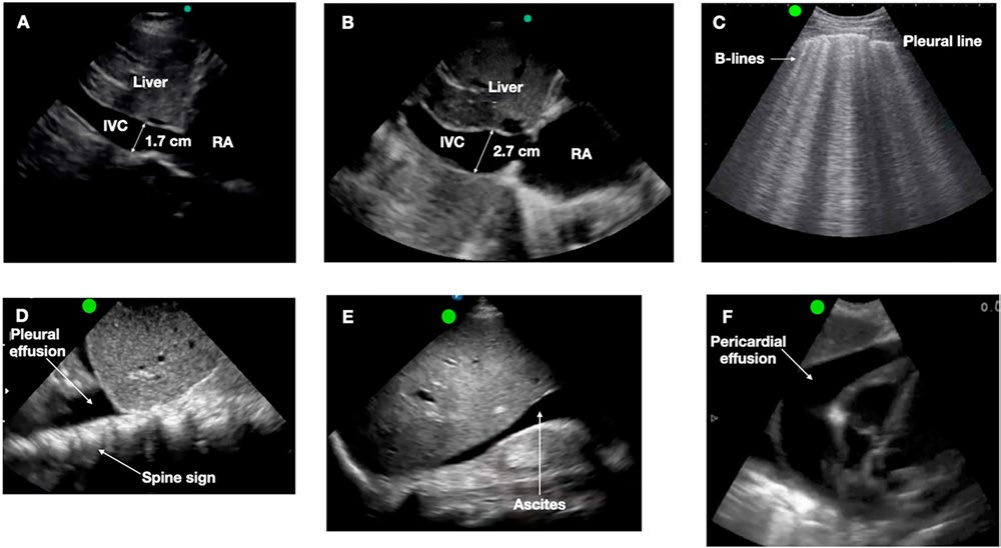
Point-of-care ultrasound for identifying volume status. Legend: A and B, ultrasound of the inferior vena cava (IVC): A, IVC < 1.7 cm, with a diameter decrease of more than 50% during inspiration, compatible with a euvolemic state; B, plethoric IVC, with a diameter of 2.7 cm and a decrease of less than 50% during inspiration, suggestive of hypervolemia; C, lung ultrasound: B-lines indicating interstitial lung syndrome, as seen in acute pulmonary edema; D, pleural effusion indicated by an anechoic image above the diaphragm; E, ascites, an anechoic image in the hepatorenal space; and F, pericardial effusion indicated by an anechoic image in the pericardial space.
